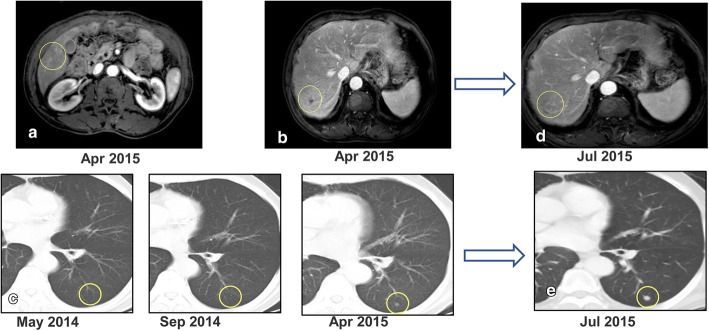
CT and MRI scans identified new lesions in the lungs and liver espectively seven months after the first-line chemotherapy and cryoablation (April 2015). a. lesion cryoablation was stable, arterial phase. b. new liver lesion. c New metastatic lesions in the lungs. c and d. The efficacy was evaluated as PD after Paclitaxel plus S-1 (second-line) chemotherapy since April 2015
